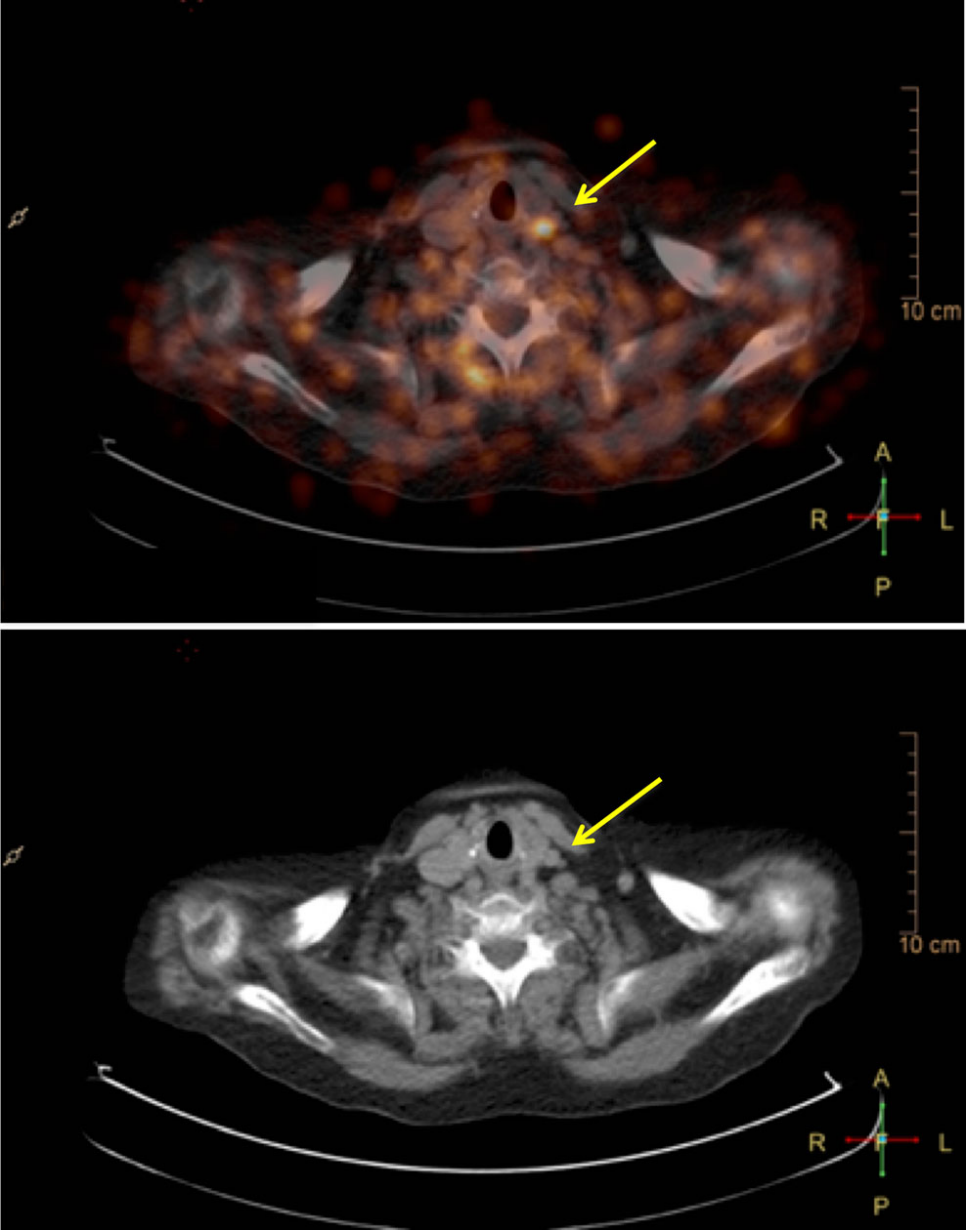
Fused PET/CT and CT images show ^68^Ga-DOTATATE uptake in the left common carotid artery close to the bifurcation (*arrows*), which is visually and semiquantitatively higher than in the right carotid artery, indicating increased inflammatory changes in the left carotid artery
